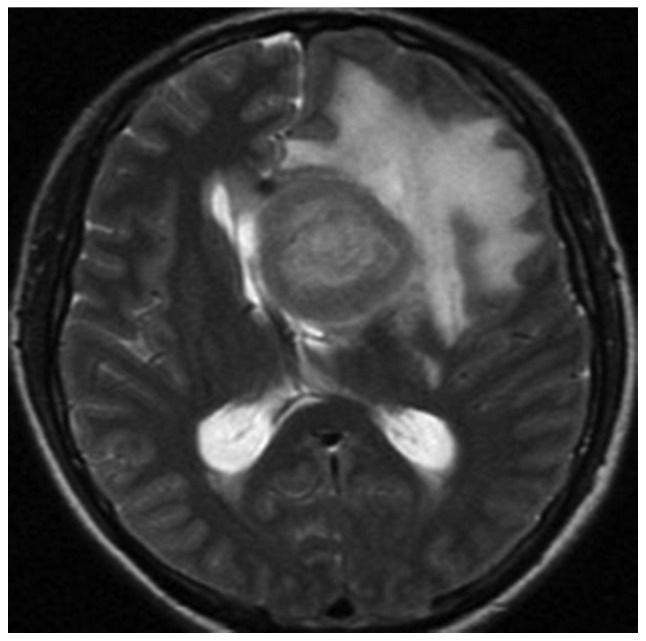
Computed tomography of the cerebrum after one year of follow-up showed a large mass in the cerebrum.
